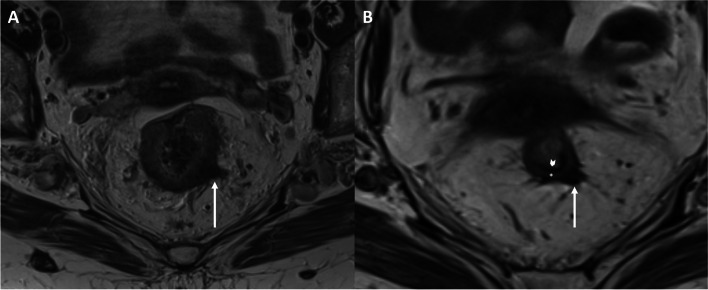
Split scar sign. A Axial T2-weighted image without fat-suppression depicting the baseline T stage, with a locally advanced rectal cancer that extends to the mesorectum at 4 o’clock (arrow). B Axial T2-weighted image without fat-suppression of the same patient after neoadjuvant therapy depicts the split scar sign, consisting of an inner hypointense mucosa (arrowhead), an intermediate signal *muscularis propria* (asterisk), and in this case, an outer hypointense layer of perirectal fibrosis (arrow)
